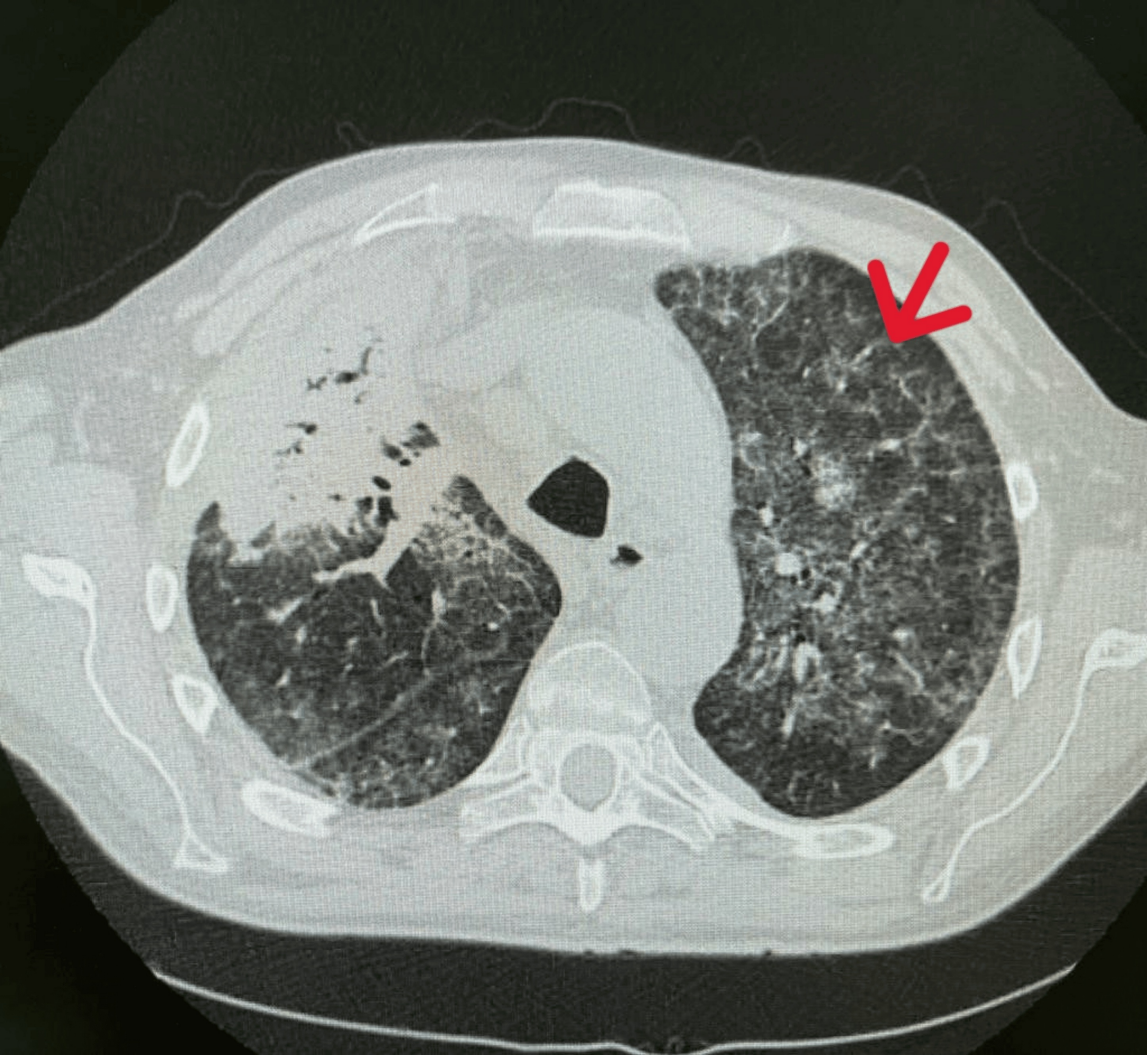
Admission chest high-resolution computed tomography (HRCT). The axial HRCT scan shows diffuse ground-glass opacities associated with interlobular and intralobular septal thickening, creating a "crazy-paving" pattern characteristic of pulmonary alveolar proteinosis. Patchy areas of consolidation are also visible in the dependent lung regions, suggesting superimposed infection.
